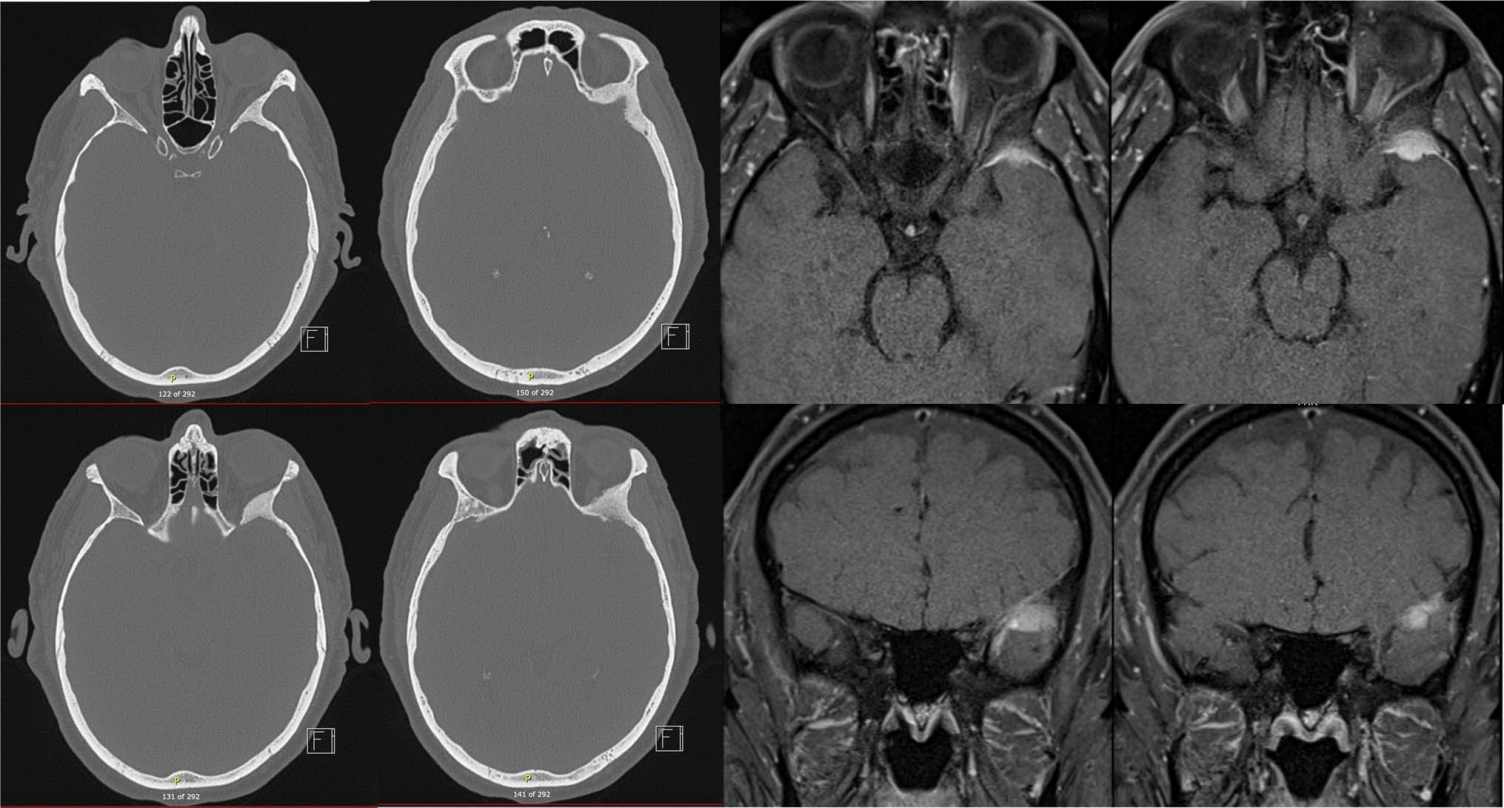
Pre-operative CT and MRI T1 with gadolinium administration demonstrating left sphenoid osseous meningioma and temporal meningioma
